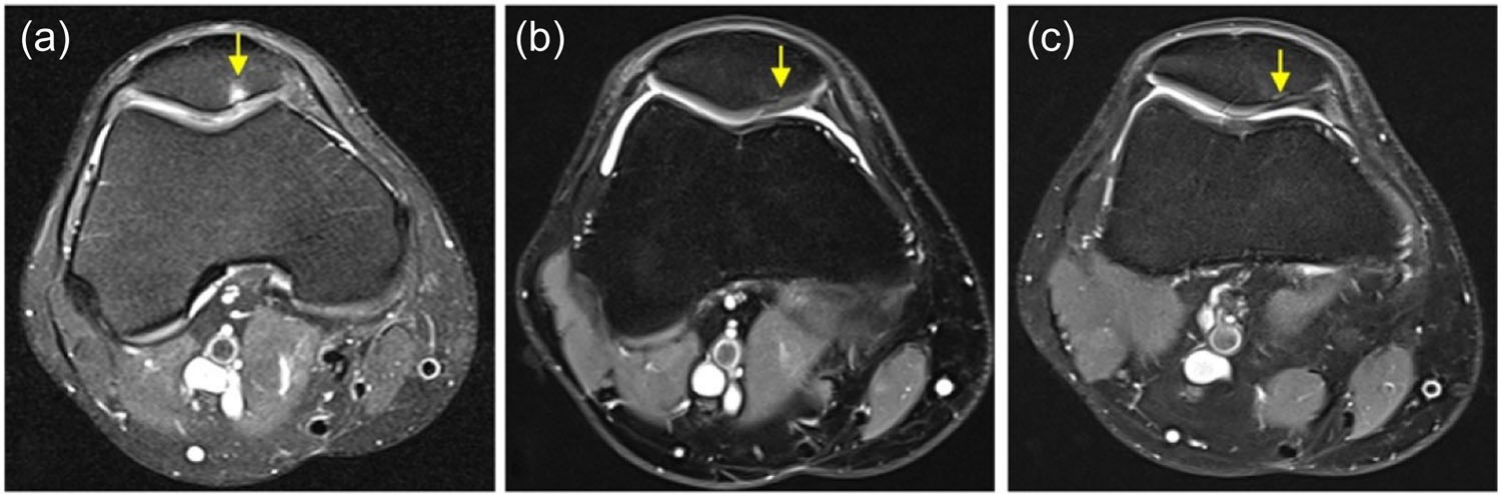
Example of good tissue regeneration, the magnetic resonance imaging (MRI) of patient #1, right knee. (a) Preoperative MRI: Focal chondral lesion of the patella grade International Cartilage Regeneration Joint Preservation Society IV located in the medial facet (arrow). (b) MRI at 2 years: No filling defect, smooth hypointense surface, complete integration of interfaces, heterogeneous hypointense signal and no bone oedema (arrow). (c) MRI at 5 years: No changes from previous MRI except for the presence of a mild focal change of the cortical plate with minimal focal bone neoformation (arrow).
